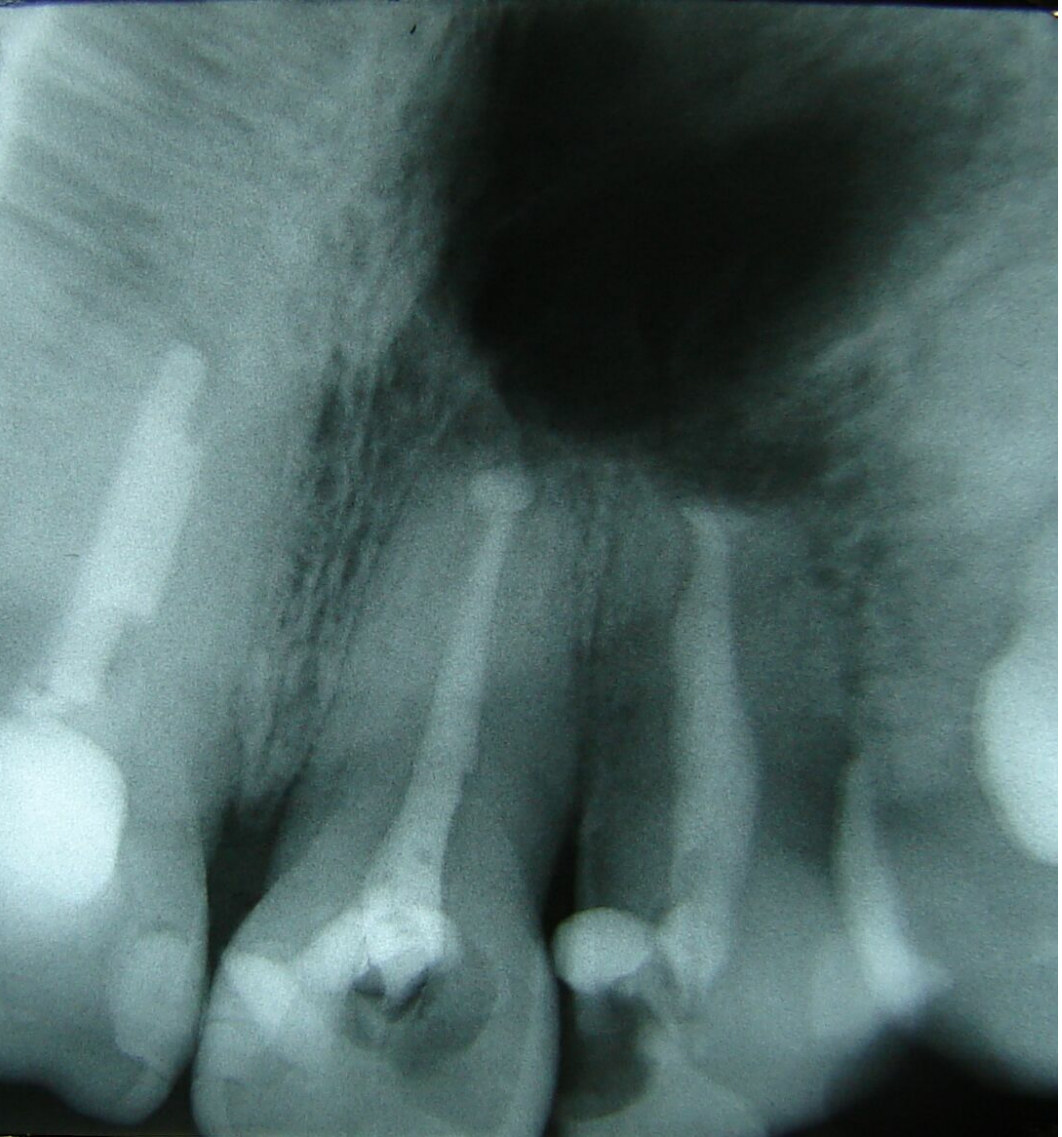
Periapical x-ray showed the filled root canals with the retrograde filling material.
